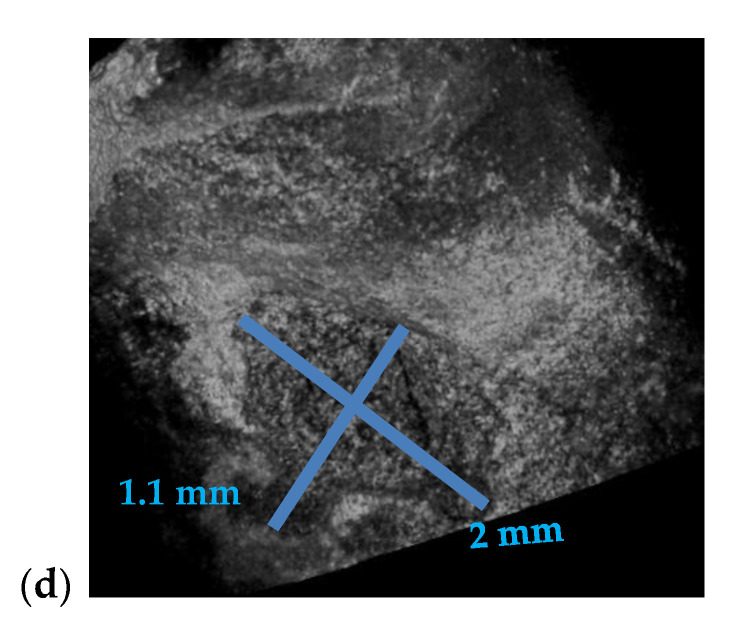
(a) A premolar tooth, with an area marked for OCT investigations; (b) section cropped from an intraoral radiograph with a view on the measured dental cavity; (c) OCT B-scan, where the depth and the width of the cavity are measured; (d) volumetric OCT reconstruction, on which the width and the length are measured.
